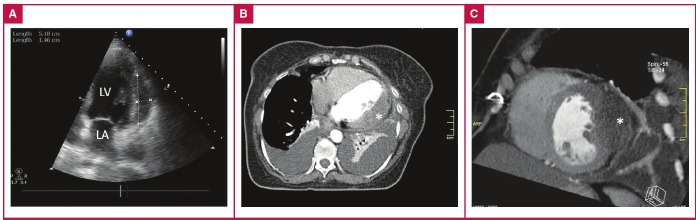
A. Transthoracic echocardiography. B, C. Axial and sagittal computed tomography sections of the heart. Intra-myocardial haematoma is seen in both echocardiography and computed tomography (asterisk). LV, left ventricle; LA, left atrium.
